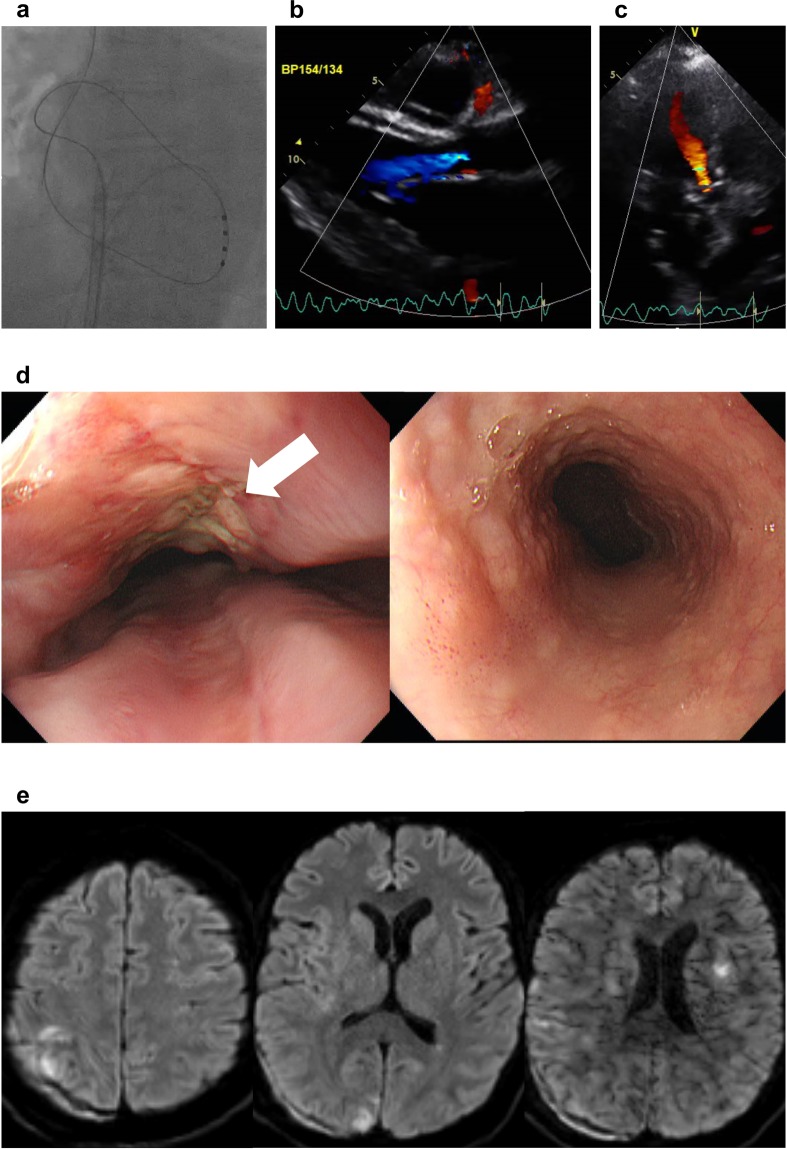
Prevention of embolic stroke and successful surgical repair. (a) Ventricular fibrillation was induced using quadripolar catheter immediately after veno-arterial extracorporeal membrane oxygenation apply. (b,c) Echocardiography revealed continuous aortic regurgitant jet suggesting complete reversal of blood flow direction in the thoracic aorta. (d) On-pump, open heart surgical repair of left atrium and esophagus was performed and follow-up endoscopy revealed no fistula. White arrow indicates operation scar. (e) Despite several small-sized embolic lesions, the patient was free of neurologic consequences.
